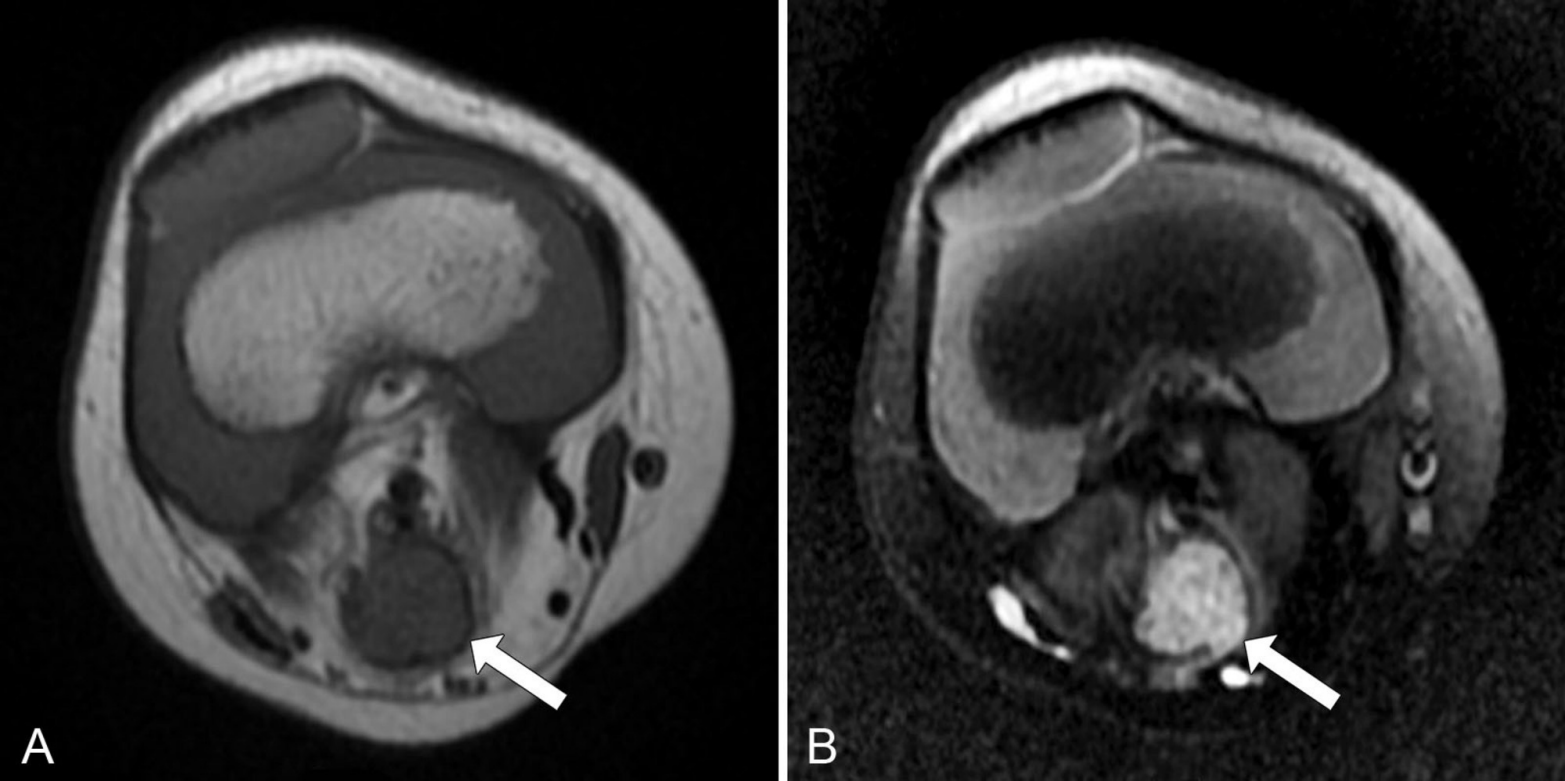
Lobulated lesion in the popliteal fossa (A) Axial T1-weighted image demonstrates a lesion in the popliteal fossa (arrow) isointense to surrounding muscle. (B) Axial proton density fat-saturated image shows that the same lesion (arrow) is heterogeneously hyperintense.
